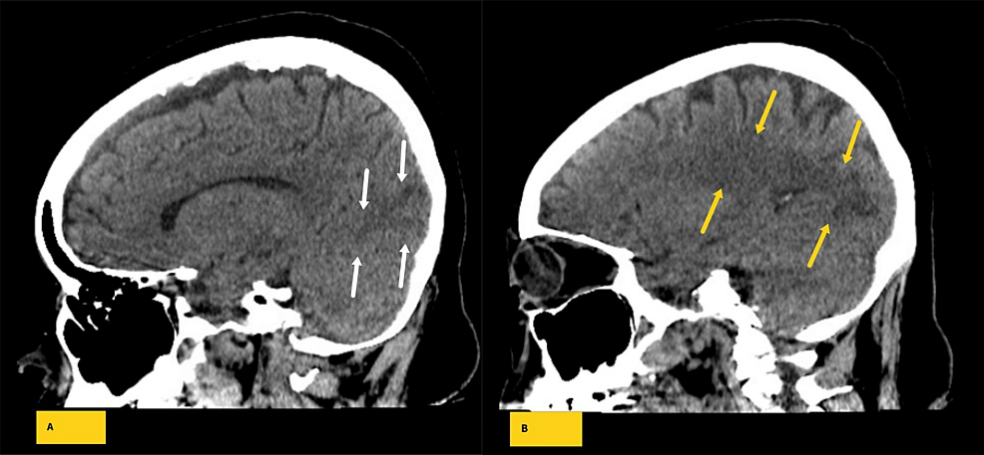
Representative sagittal computed tomographic images of the brain demonstrate hypodensities in the left (B - yellow arrows) greater than right (A - white arrows) parieto-occipital lobes raising the possibility of posterior reversible encephalopathy syndrome.
